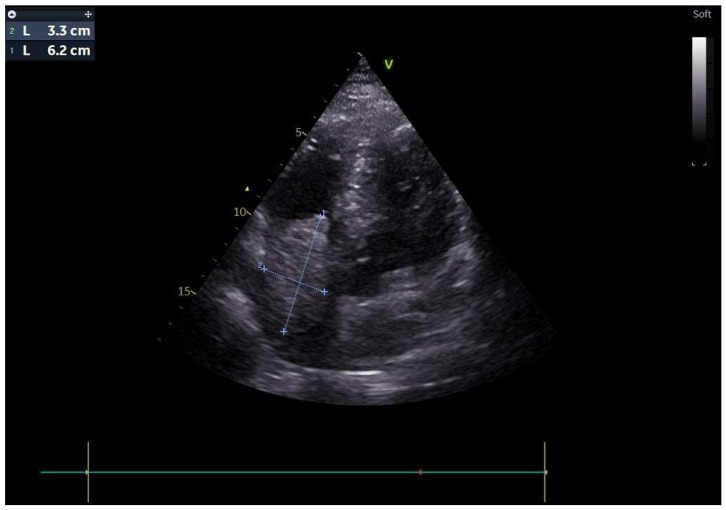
Transthoracic echocardiography (TTE) showed of a low-moving hypoechoic thrombus or mass.
